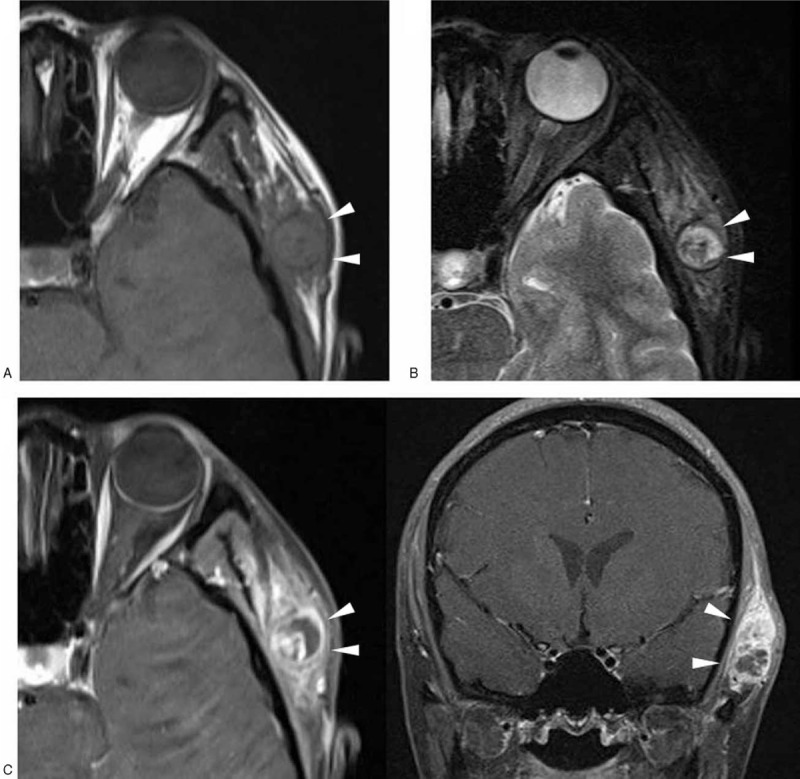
Magnetic resonance images reveal an ill-demarcated soft tissue mass (arrowheads) located in the temporal scalp between the temporal fascia and the temporalis muscle, exhibiting a homogeneous hypo- to isointense signal on a T1-weighted image (A); a heterogeneous mixture of iso- and hyperintense signals on a T2-weighted image (B); and heterogeneous intense enhancement with peritumoral infiltration-like signal changes along the adjacent soft tissues (including the temporal fascia and the superficial portion of the temporalis muscle) on fat-suppressed T1-weighted images taken after intravenous administration of gadolinium (C).
